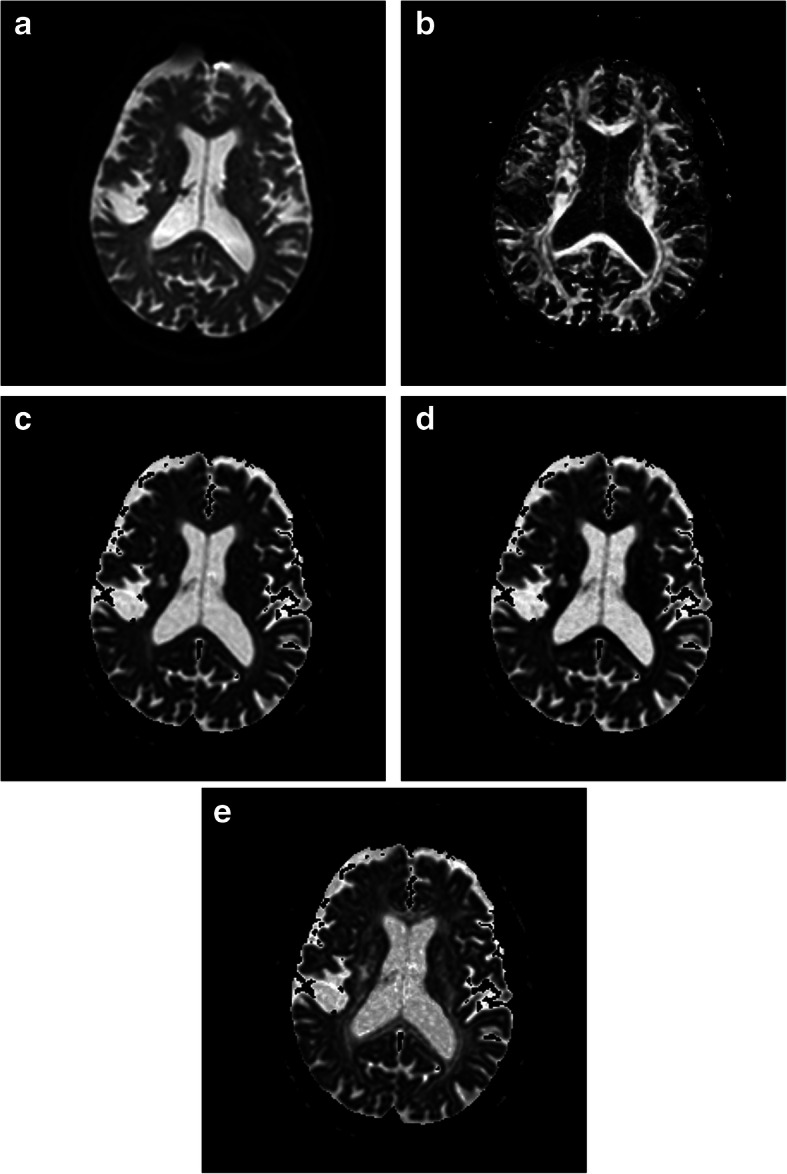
The b0 of the DWI (a) of a 75 years old male showed WMH (Fazekas scale 1) in the pFL, pOL, pPL and dCO. FA (b) of WMH in the pFL are lower than that of pOL, pPL and dCO. MD (c) and RD (d) of WMH in the pFL are higher than that of pOL, pPL and dCO. AD (e) of WMH in the pFL are close to that of pOL, pPL and dCO
